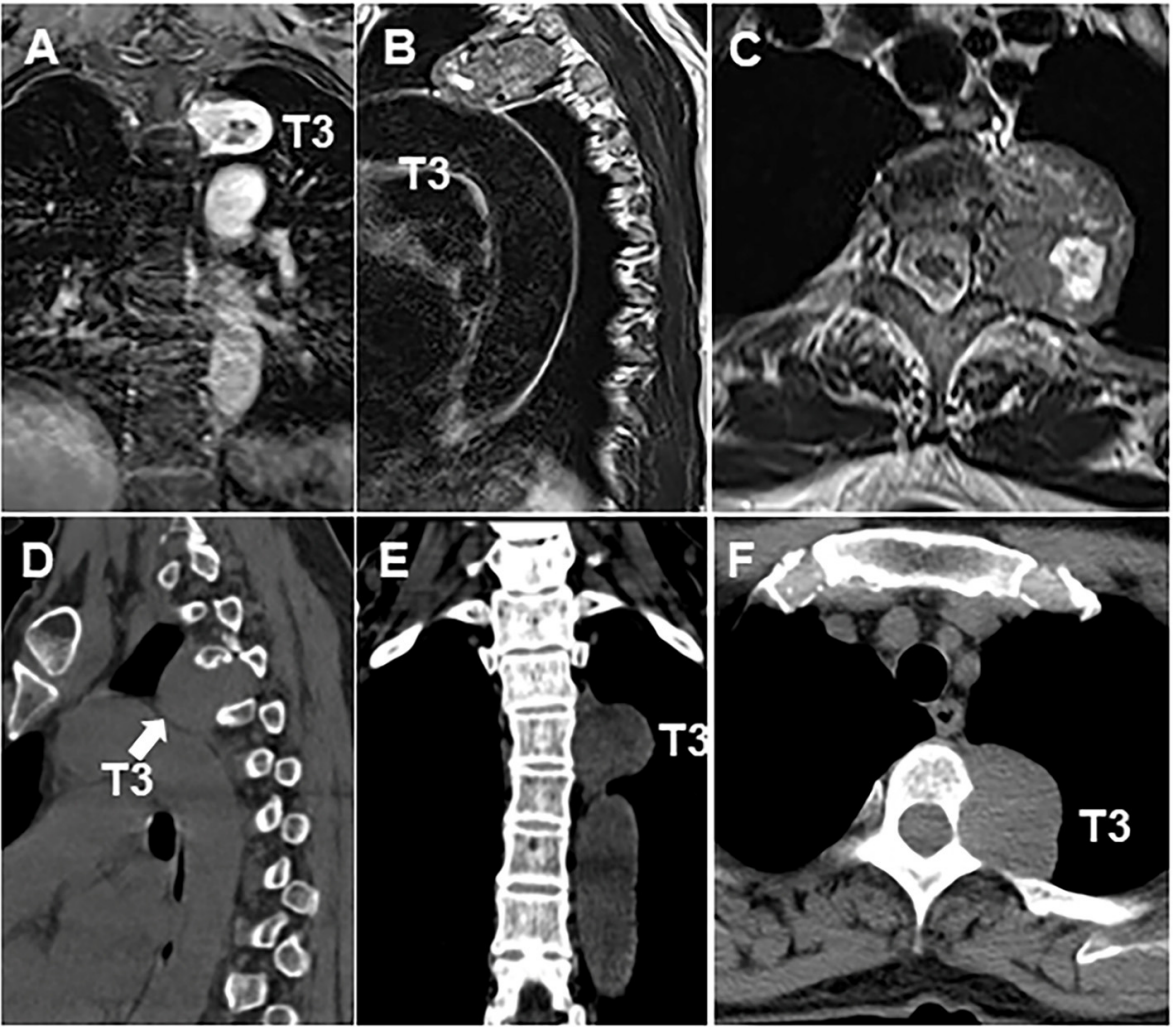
Thoracic MRI and CT scan showed the size of the lesion to the left of the T3 (A–F) vertebral body was ~3.7 × 5.3 cm. Arrow indicates the location of tumor.
